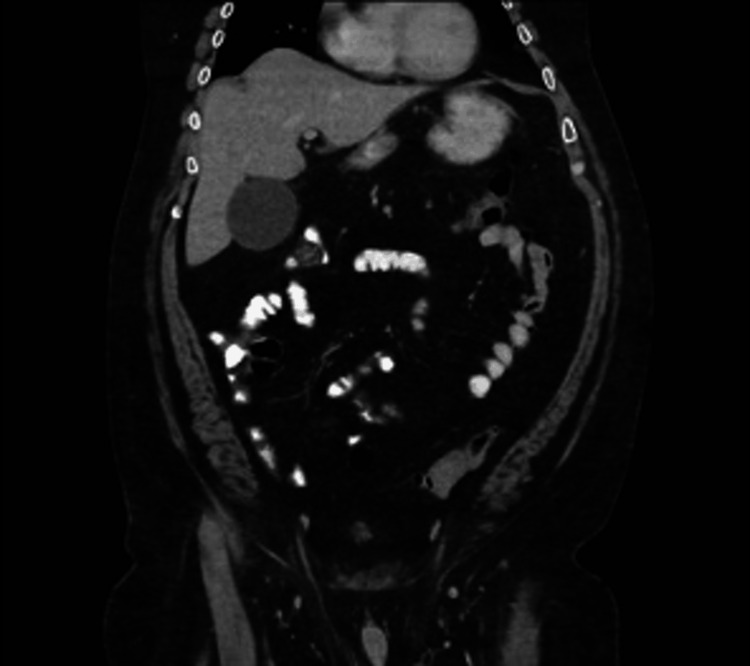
Coronal CT after decompression of gastric volvulus
